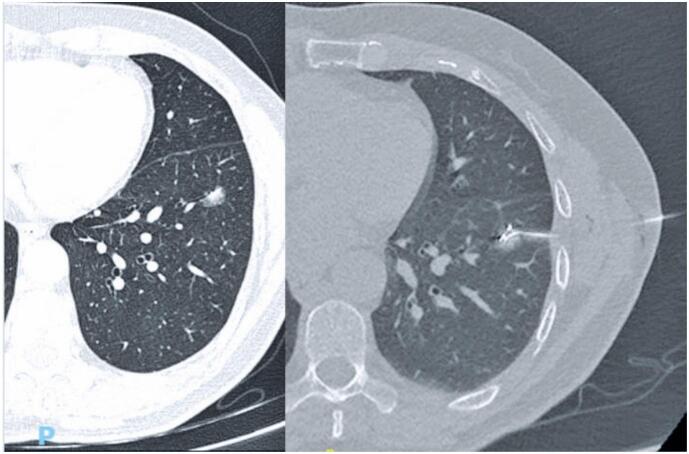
66-year-old female patient with previously resected lung cancer. Follow-up CT shows a new small nodule in the left lower lobe. The lesion was preoperatively marked with the metallic spiral wire and resected. Pathology confirmed invasive lepidic adenocarcinoma
